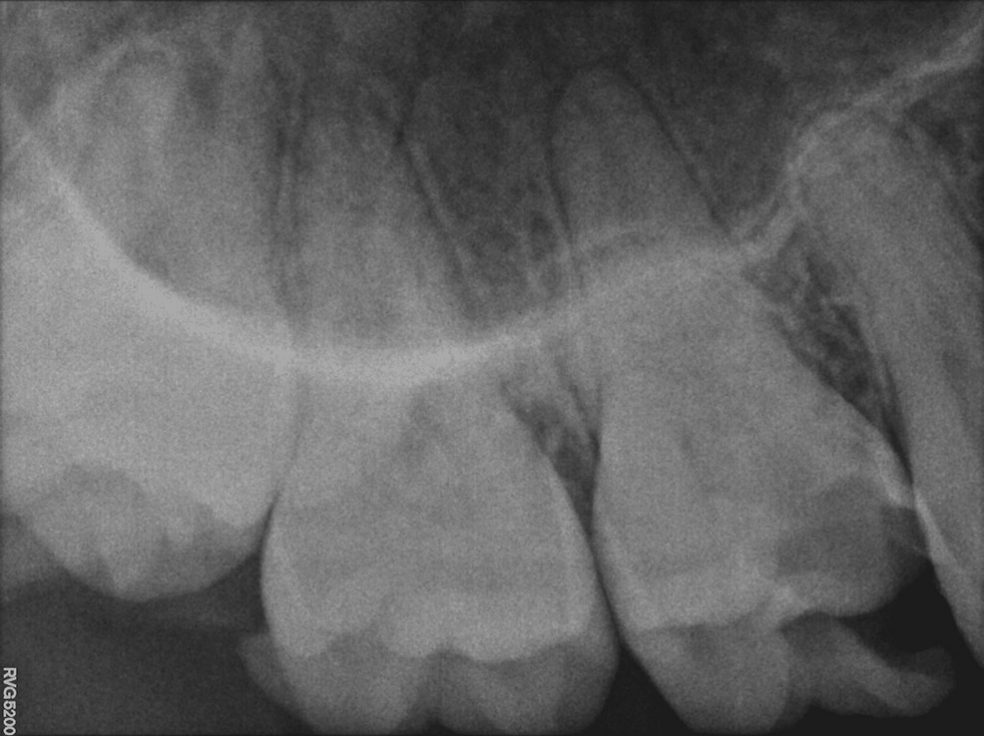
Preoperative radiograph.
